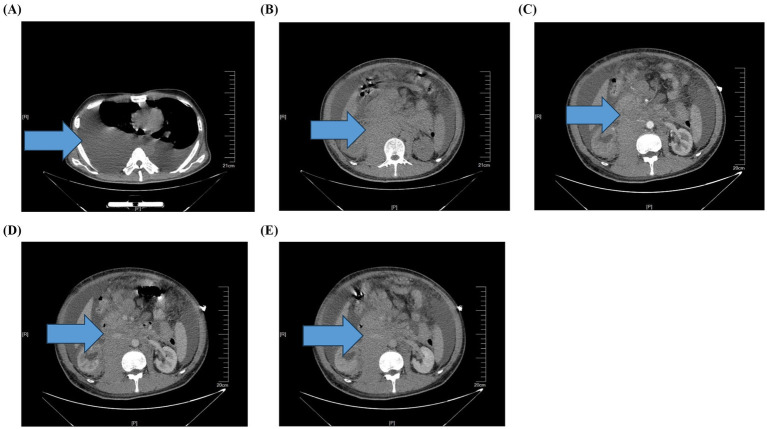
(A) Imaging findings: chest CT (mediastinal window) showing bilateral pleural effusions (arrows). (B) Imaging findings: abdominal CT plain scan: large retroperitoneal mass (arrow). (C) Imaging findings: arterial phase: minimal enhancement of the mass (arrow). (D) Imaging findings: venous phase: persistent low enhancement (arrow). (E) Imaging findings: delayed phase: heterogeneous enhancement (arrow).
